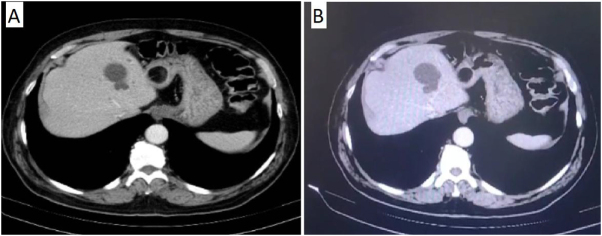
A contrast-enhanced abdominal CT scan showing a well-defined submucosal mass in the distal stomach, with no evidence of metastatic disease.
